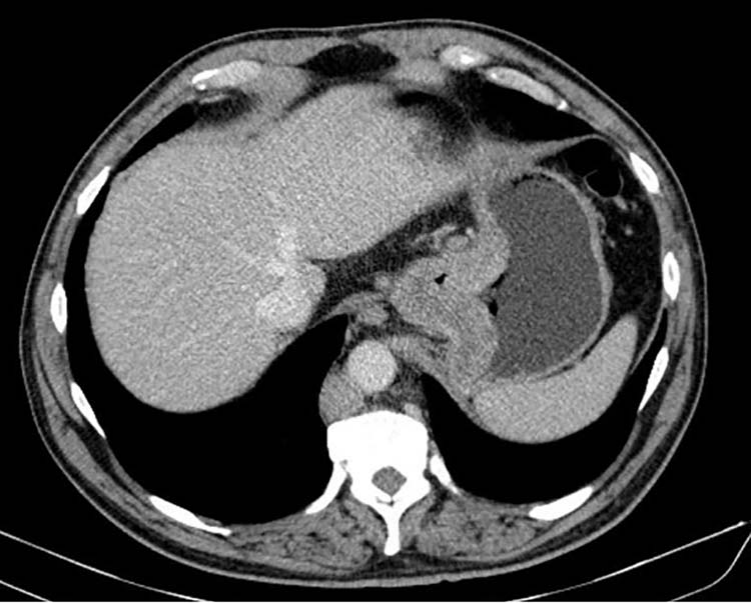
CT scan showing exuberant parietal thickening of the cardia.
